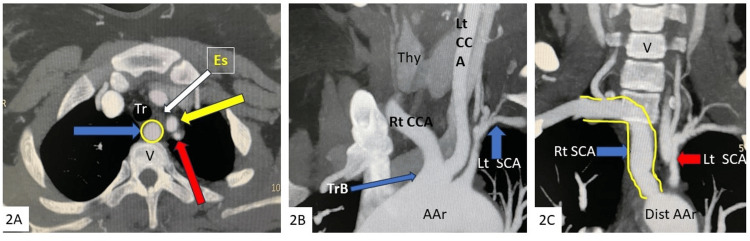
Axial (A) and anterior (B) to posterior (C) coronal MIP images from CT angiography showing aberrant right subclavian artery with prevertebral/retroesophageal course, and having origin from aortic arch distal and posterior to left SCA. Right SCA encircled and indicated by the blue arrow in the axial image (A) and outlined in the coronal image (C). Also noted common trunk origin of the bilateral common carotid artery SCA: subclavian artery; CCA: common carotid artery; Aar: aortic arch; TrB: truncus bicaroticus; Tr: trachea; Es: collapsed esophagus; Thy: thyroid gland, reference for anterior location; V: vertebra, reference for the posterior location
